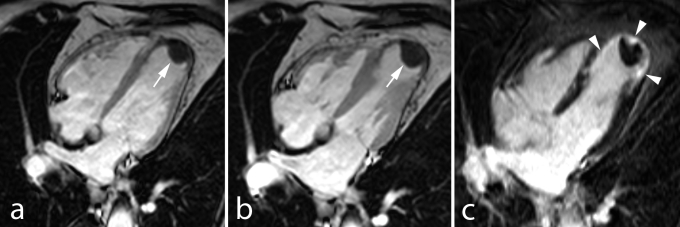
Old anterior MI in 75 year old man presenting with heart failure symptoms. Horizontal long–axis cine MRI (a) and early (b) ce–MRI show apical aneurysm with large typically hypoenhanced thrombus (*arrows*, a,b). Late ce–MRI in the same plane shows apical transmural enhancement (*arrowheads*, c).
